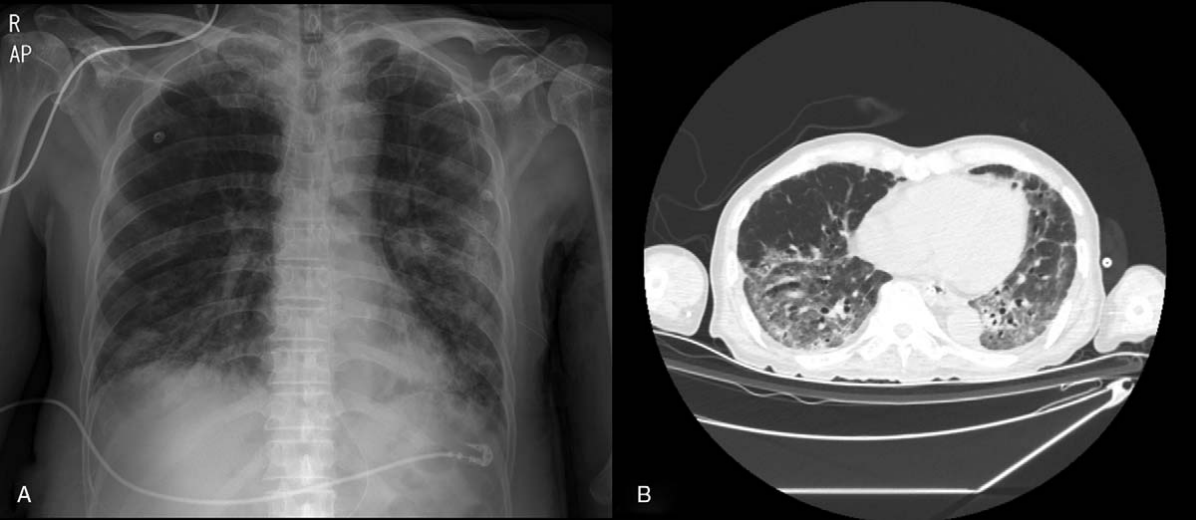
CXR and CT scan revealed diffuse viral pneumonia associated with COVID-19. A, CXR. B, CT scan. CXR = Chest X-ray.
